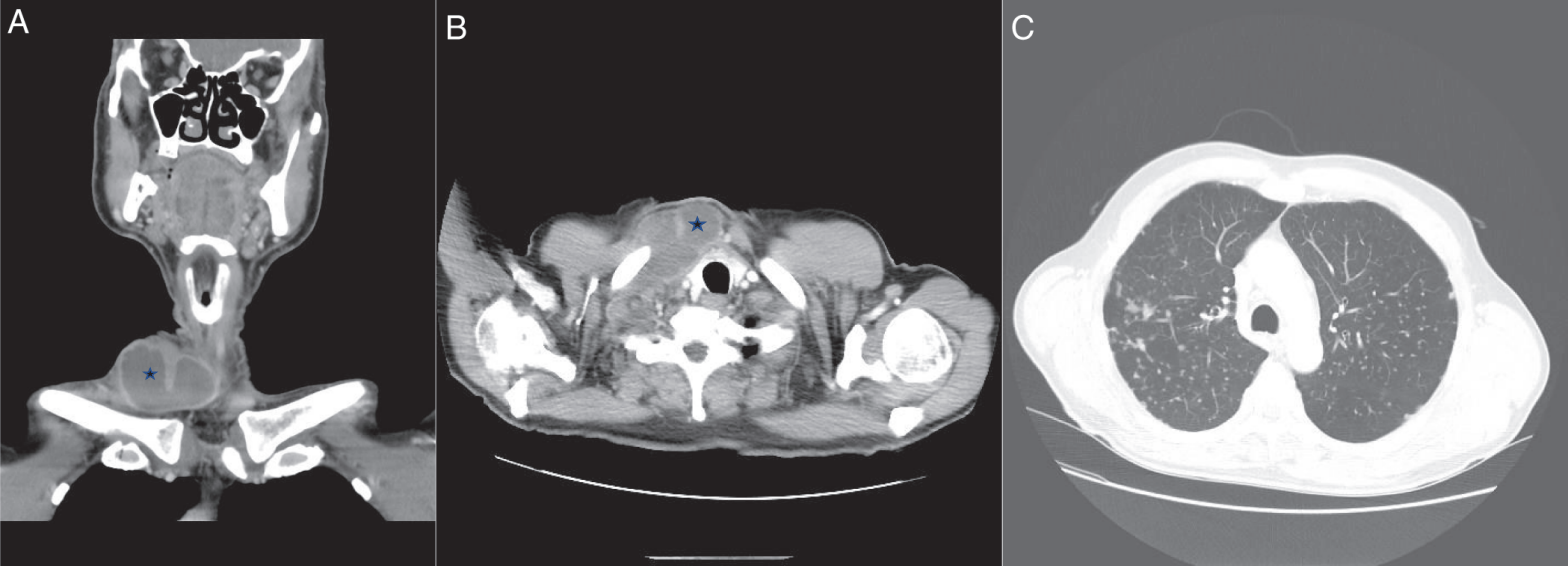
(A,B) Axial and coronal computed tomography (CT) scans of right lower neck cystic mass with peripheral enhancement. (C) CT thorax showing multiple well‐defined pulmonary nodules in bilateral upper lobe predominance.
